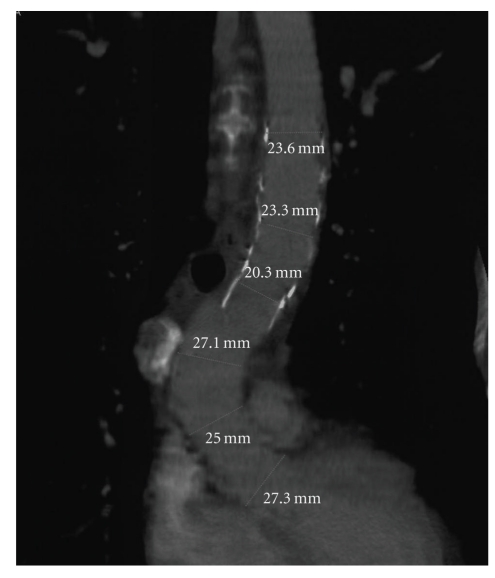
Angio-CT scan two years after endovascular repair.
